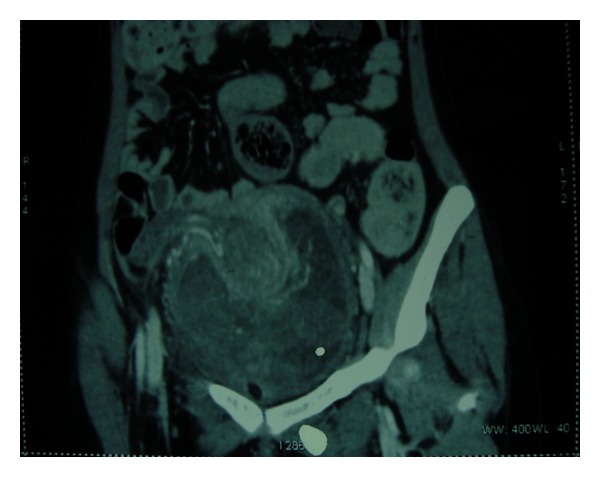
Coronal section computed tomography showing heterogeneously enhancing mass lesion engulfing the uterus, broad ligament and accompanying vessels.
